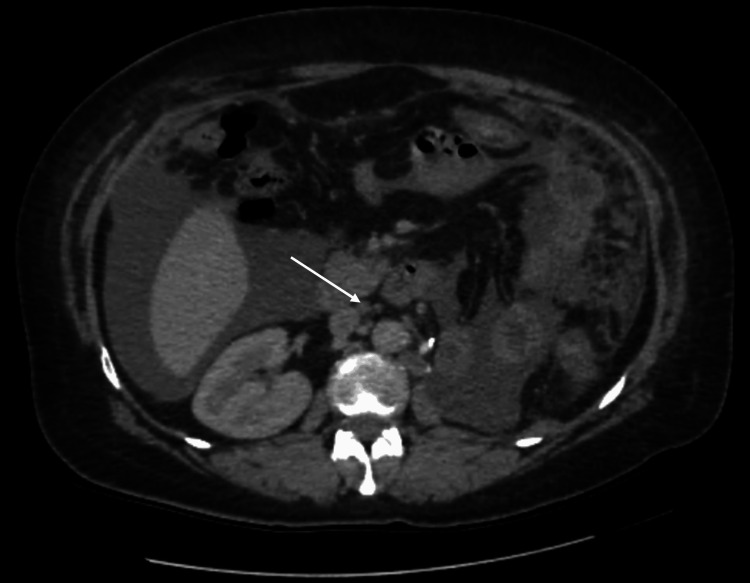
Axial CT scan showing latero-aortic lymph nodes
